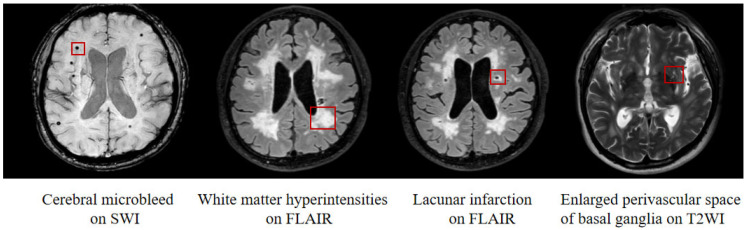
Neuroimaging features of cerebral small vessel disease. From left to right, the images show cerebral microbleed on SWI, white matter hyperintensities on FLAIR, lacunar infarction on FLAIR, and enlarged perivascular space of basal ganglia on T2WI. Abbreviations: SWI: susceptibility weighted imaging; FLAIR: fluid-attenuated inversion recovery imaging; T2WI: T2-weighted imaging.
